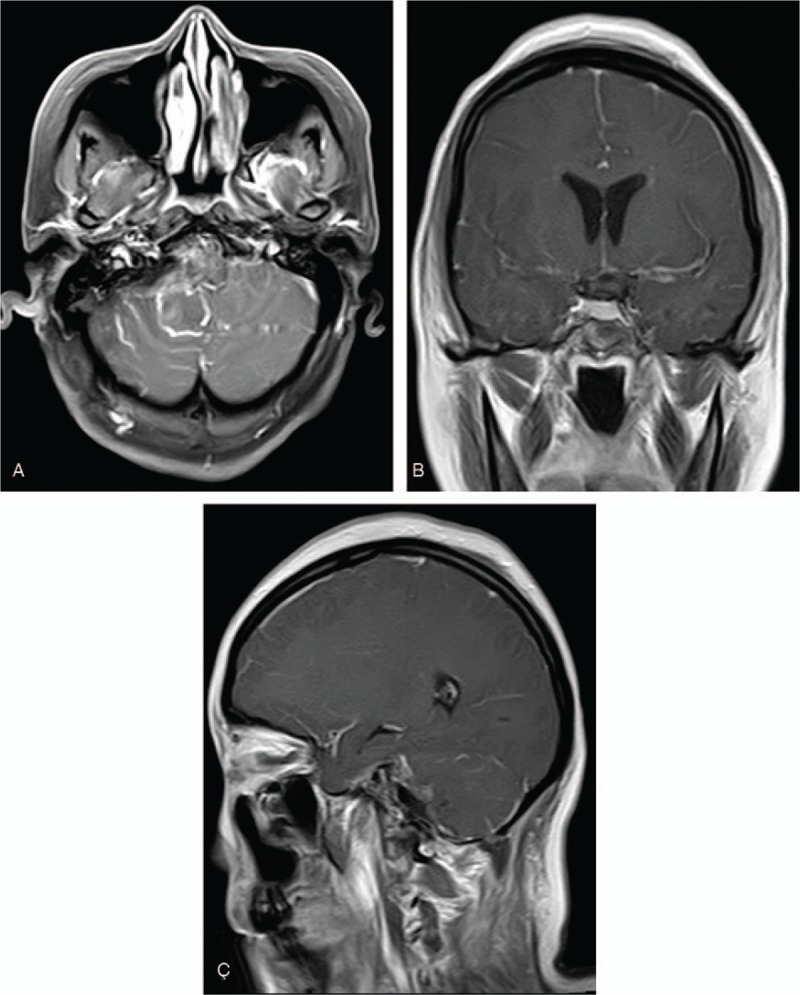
(A–C) Postoperative MRIs showing total resection of the tumor. MRIs = magnetic resonance imaging.
